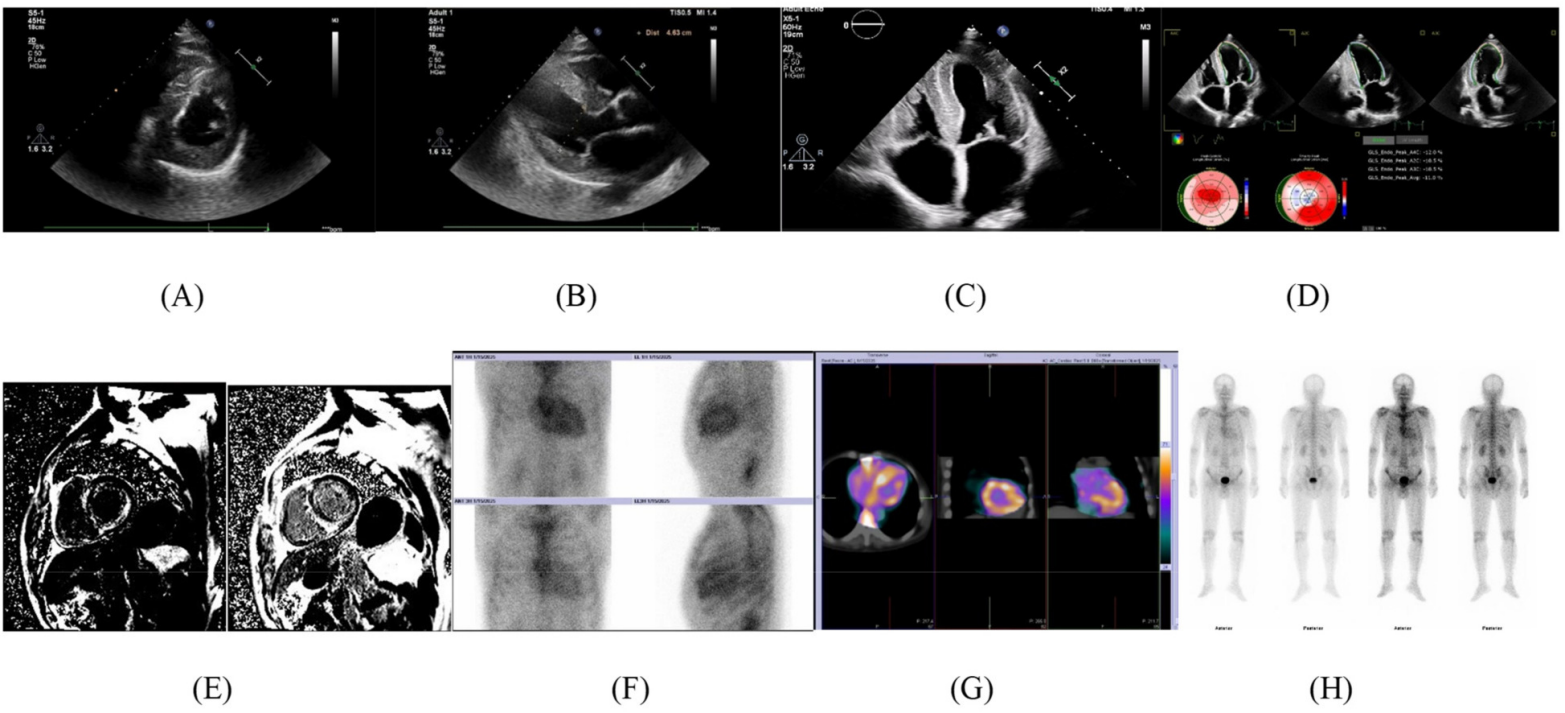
Imaging findings. (A) Fine punctate or granular echo enhancement in the interventricular septum. (B) Uniformly thickened myocardium with a ground-glass appearance. (C) Bilateral atrial enlargement. (D) Left ventricular longitudinal strain bullseye pattern/apical sparing phenomenon/strawberry sign. (E) MR: delayed enhancement shows “dust-like” enhancement in the left ventricle, right ventricle, left atrium, and interatrial septum. (F) ① Heart anterior and lateral views at 1 h and 3 h: The cardiac radiopharmaceutical shows diffuse increased uptake, while the ribs are poorly visible; ② Heart-to-lung ratio (H/CL) for the contralateral lung: 1 h: 1.77, 3 h: 1.51 (1 h H/CL > 1.5 is considered positive, 3 h H/CL > 1.3 is considered positive). ③ Visual score: 3 points (myocardial uptake is greater than rib uptake, with mild or no rib uptake). (G) SPECT/CT fusion imaging: Diffuse radiopharmaceutical uptake is mainly in the ventricular walls. (H) Whole-body imaging: Increased uptake in the heart, spine, kidneys, and bladder.
